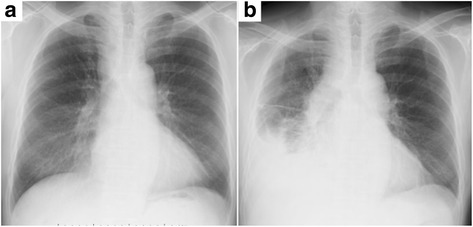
Chest radiographs after EBUS-TBNA. a Chest radiograph 1 day after EBUS-TBNA. b A chest radiograph 9 days after EBUS-TBNA showing enlargement of the mediastinum, increased pleural effusion, and an infiltrative shadow in the right lower lung field
